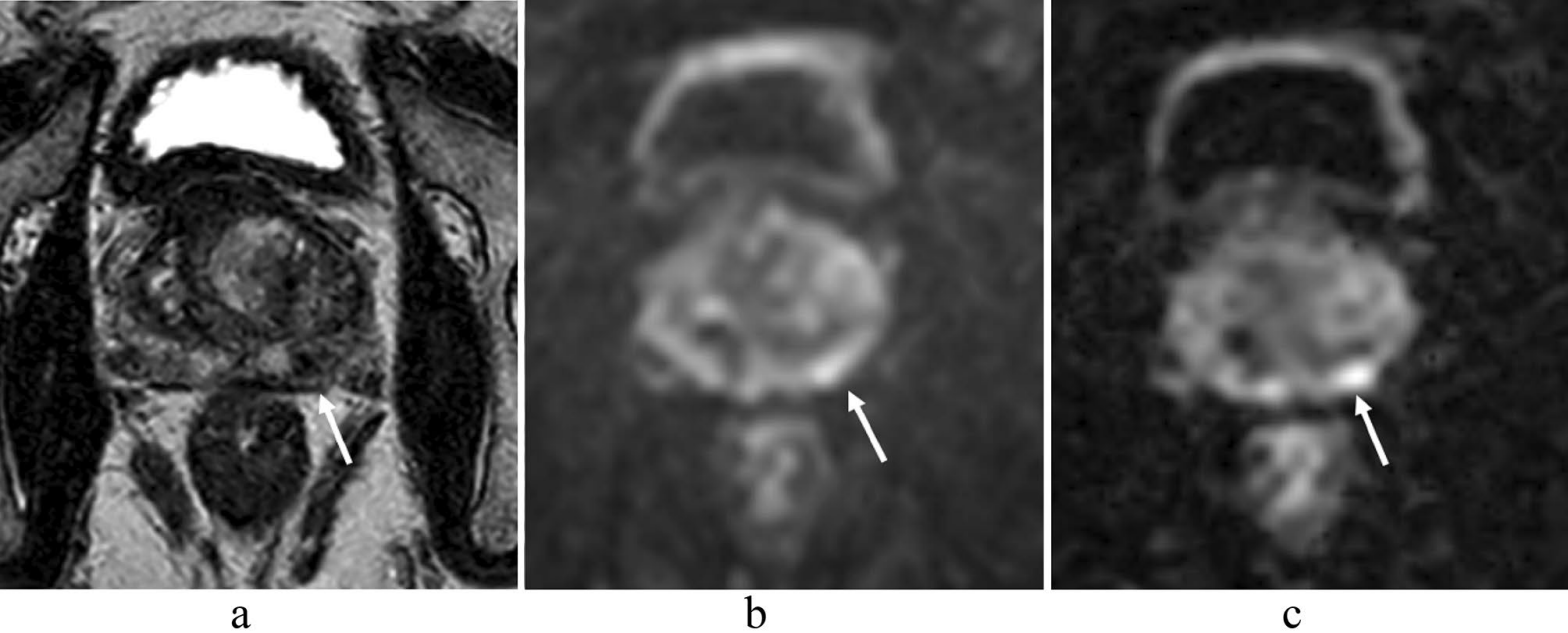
A 58-year-old man with prostate cancer (PSA level, 9.43 ng/mL; Gleason score, 4 + 3) in the left peripheral zone. A homogeneous hypointense lesion is seen on T2-weighted imaging (a) (arrow). A focal hyperintensity is depicted clearly on msEPI DWI (c) (arrow). Lesion conspicuity is lower in ssEPI DWI (b) than in msEPI DWI (c) (arrow). *PSA* prostate-specific antigen, *msEPI* multi-shot echo-planar imaging, *DWI* diffusion-weighted imaging, *ssEPI* single-shot echo-planar imaging.
